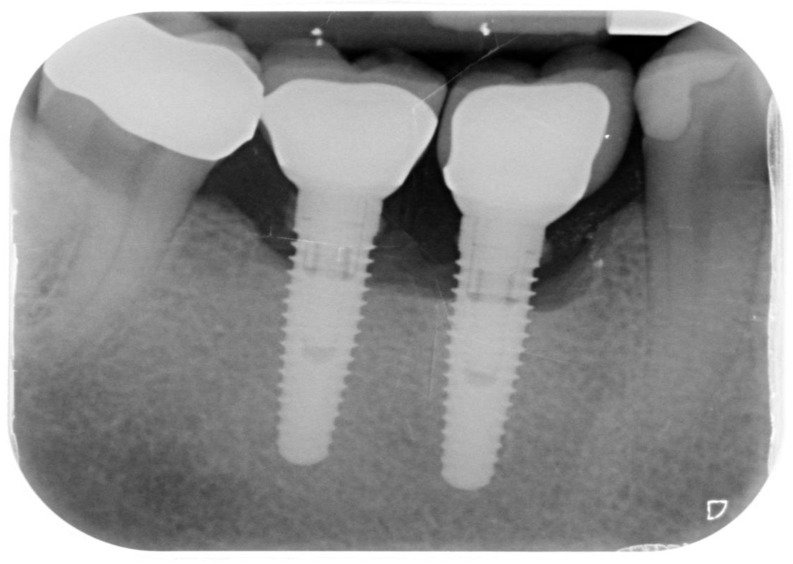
Periapical radiograph of implants affected by peri-implantitis in the lower right quadrant.
